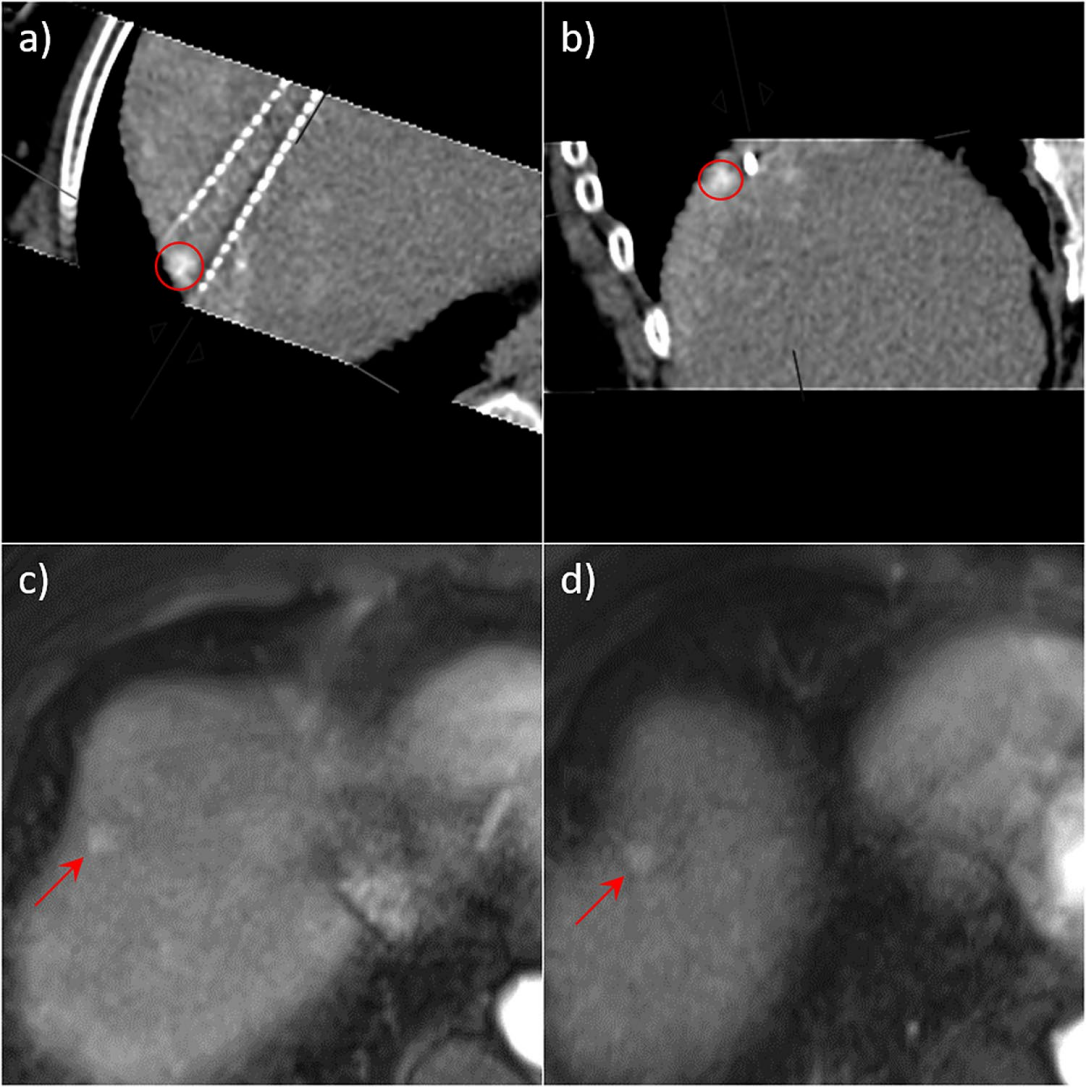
Another example of an IRE with residual tumor after ablation with 2 needles. (a) and (b) show MPRs of the CT with needle placement immediately before ablation, (a) in parallel alignment to the needle plane, (b) orthogonal to it. HCC, marked with lipiodol the day before, is outlined as red circle. In this case the depth of the lateral needle was insufficient due to the subcapsular location of the tumor (Ndep = 11 mm), the needle divergence was borderline high (Ndiv = 5.7°) and the tumor-center-to-ablation-center distance was too high (TACD = 8 mm). (c) Contrast-enhanced MRI before ablation with hyperarterialised HCC (arrow). (d) Residual tumor 6 weeks after ablation.
